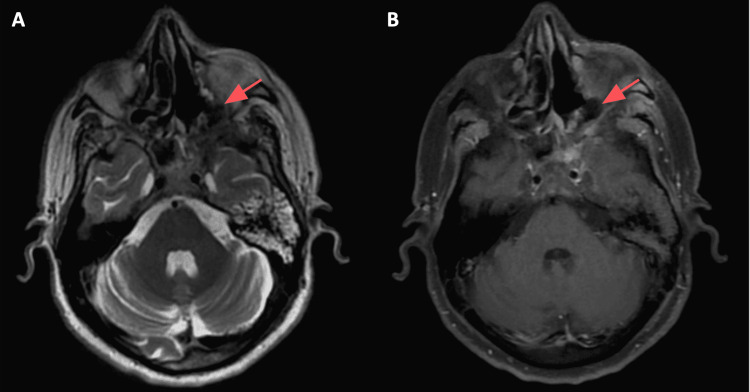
Postoperative MRI images: axial T2 (A) and axial T1 (B) with fat saturation showing resolution of the mucormycosis infection (red arrows showing the site of the previous mucormycosis infection).
